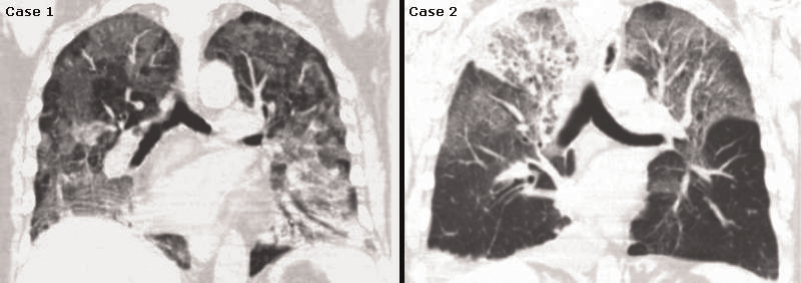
CT scan findings in DAH, Sagittal high resolution chest CT without contrast showing bilateral ground glass opacities with interstitial septal thickening suggestive of acute interstitial process.
